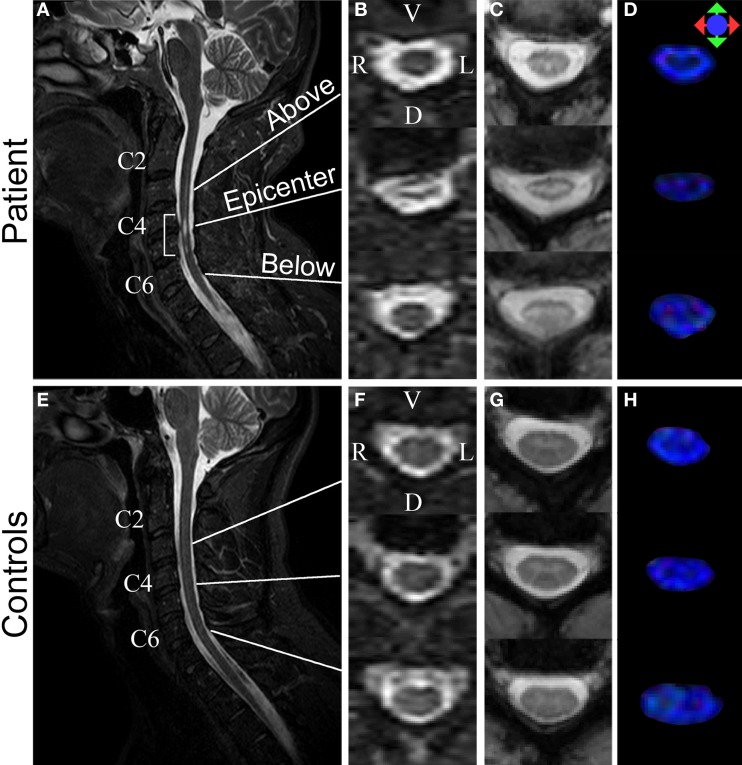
Structural MR images acquired from the patient (top row) and a control (bottom row). (A,E) Sagittal T2-w images. Bracket in (A) highlights injury epicenter of the patient. (B,F) Axial T2-w images. (C,G) Axial MTCSF images. (D,H) Axial FA color maps. The FA maps are color-coded using a standard diffusion color-encoding scheme (r/l: red, a/p: green, s/i: blue). Each row of (B–D) shows a section from: (1) above the injury epicenter, (2) injury epicenter, and (3) below the injury epicenter. Images from the corresponding cervical levels in the control are shown in each row of (F–H).
